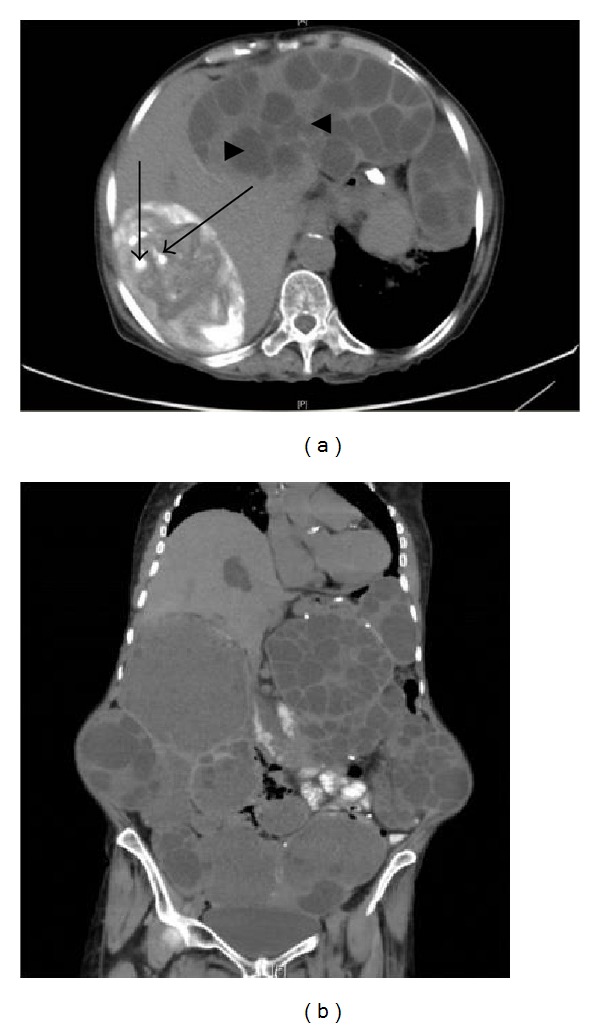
(a) CT scan abdomen showing multiple cystic lesions of variable appearance and size, with partial calcification “long arrows” and intrahepatic daughter cyst “arrow head.” (b) Multiple cystic lesions of variable appearance and size with septa and wall trabeculation.
